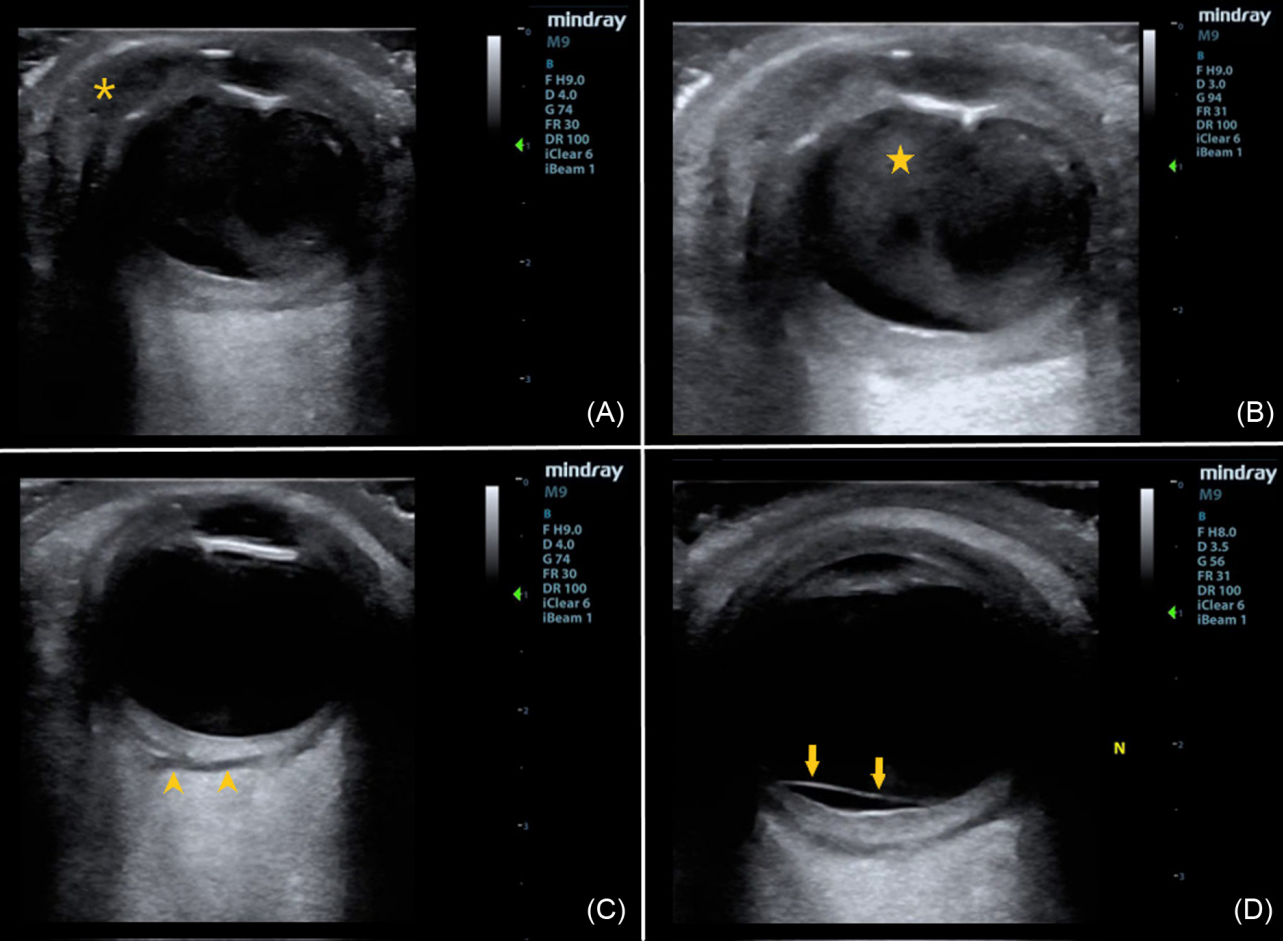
(A) The hypoechoic fluid collection (asterisk) in the lateral aspect of the orbit is consistent with orbital abscess. (B) The echogenic purulent debris within the vitreous humor (star) has an irregular and loculated appearance and is the key ultrasonographic finding of endophthalmitis. (C) and (D) The hypoechoic region posterior to retina and choroid (arrowheads) represents fluid in sub-Tenon’s space (potential space), and the thin membrane crossing the optic nerve (arrows) represents a posterior vitreous detachment, both of which are less common ultrasonographic findings of endophthalmitis.
